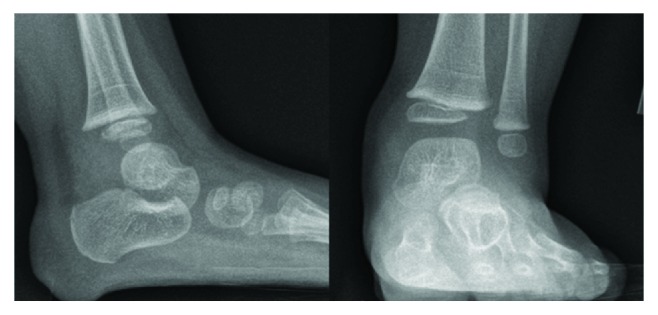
Lateral and AP plain films one year after I&D.
